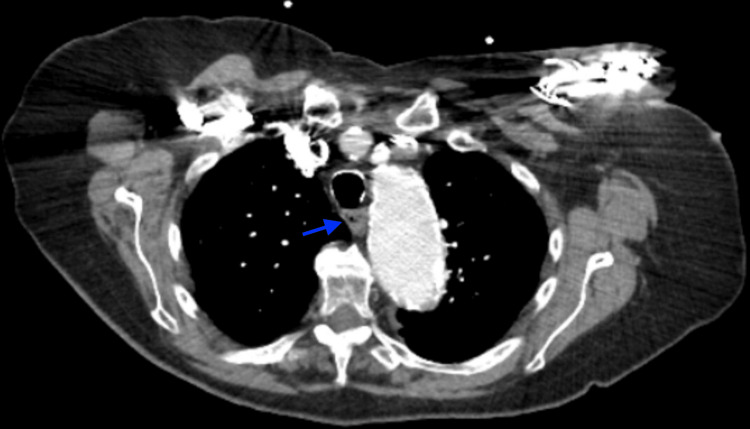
CTA in axial view The blue arrow points to a compressed esophagus by an aortic aneurysm. CTA: computed tomography angiography
